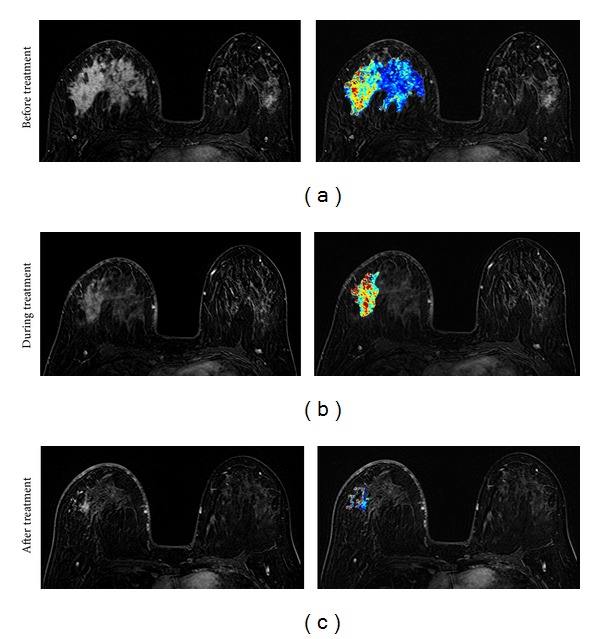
A 31-year-old patient with non-mass-like enhancement lesion (invasive ductal cancer with extensive carcinoma in situ components). From (a) to (c), the contrast-enhanced (subtraction) images selected from the same level in pretreatment, F/U-1, and F/U-2 MRI are shown. The tumor boundary cannot be clearly determined, and thus the extent of the tumor cannot be measured precisely. The area of the enhanced tumor tissues and the degree of enhancement are decreasing with treatment, indicating a good response to the chemotherapy. The right panel shows the corresponding color-coded *K*
